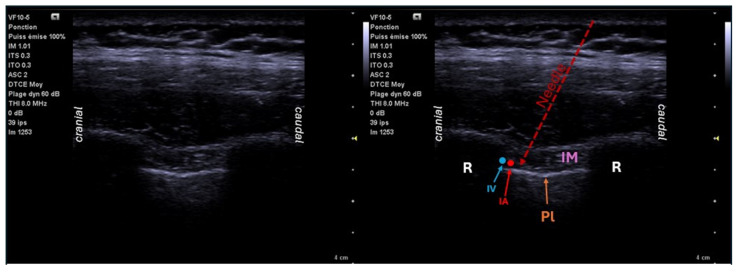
Ultrasound location for intercostal block: sagittal view T4–T5. R: rib; Pl: pleura; IM: intercostal muscle; IV: intercostal vein; IA: intercostal artery.
